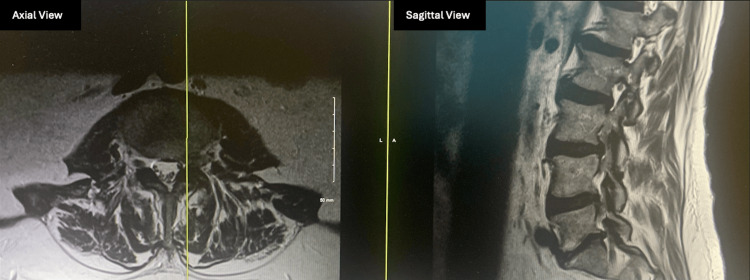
Postoperative MRI axial and sagittal views of the left spine at the area of left-sided stenosis MRI: magnetic resonance imaging
